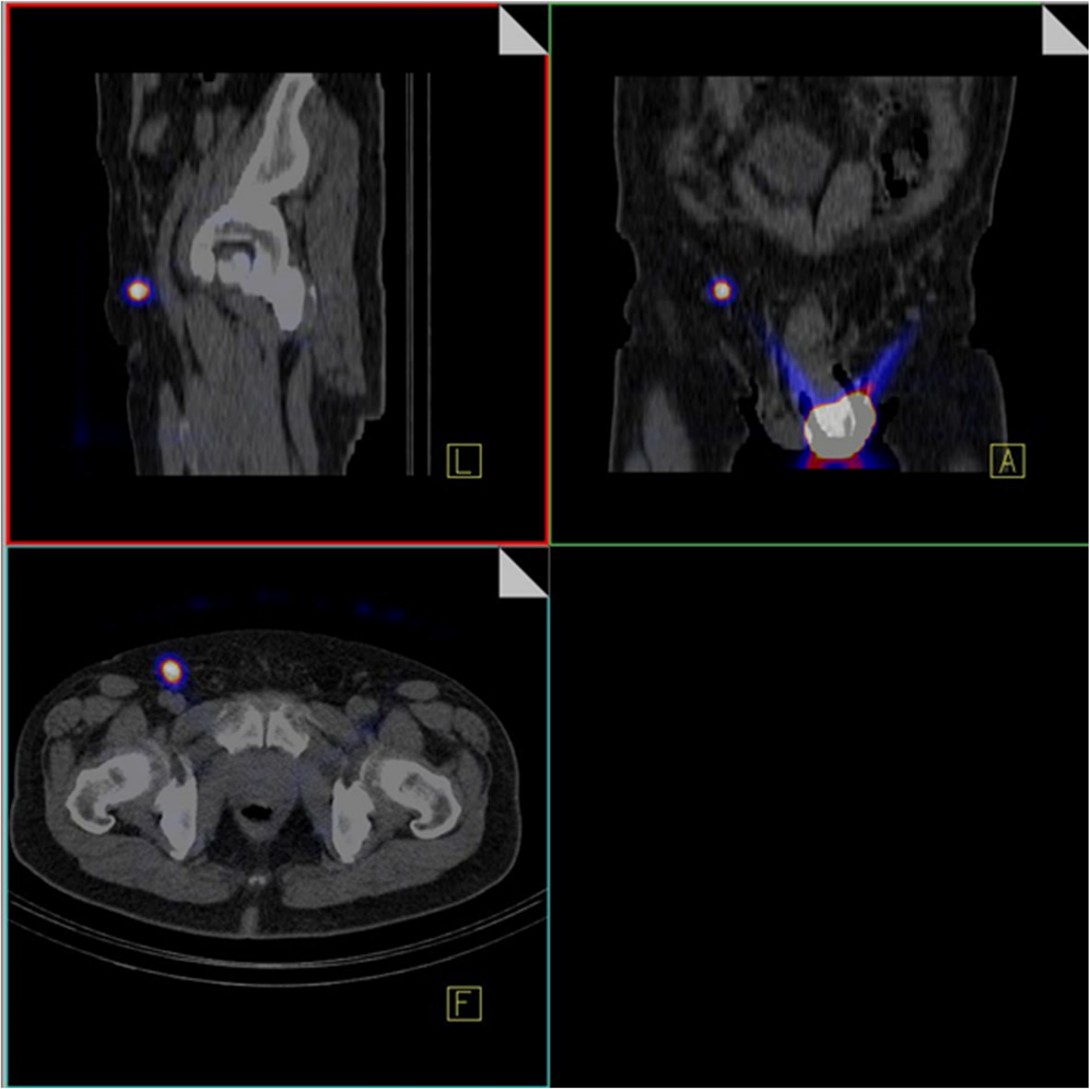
Preoperative visualization of sentinel lymph nodes via SPECT/CT: Evidence of so-called “hot spots” in the right inguinal region as well as in the periphery of the tumor (application site)
